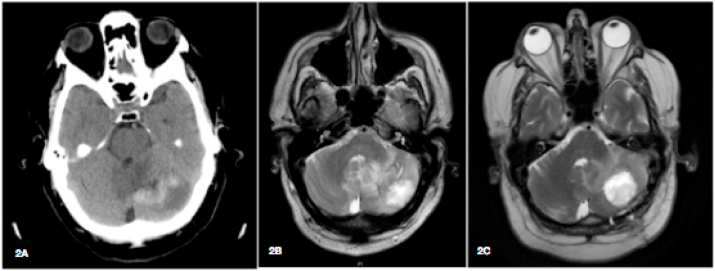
2A: Brain CT scan on day +7. 2B: Magnetic resonance imaging on day +10. 2C: Magnetic resonance imaging on day +100 (recurrence after resection on day +81).
